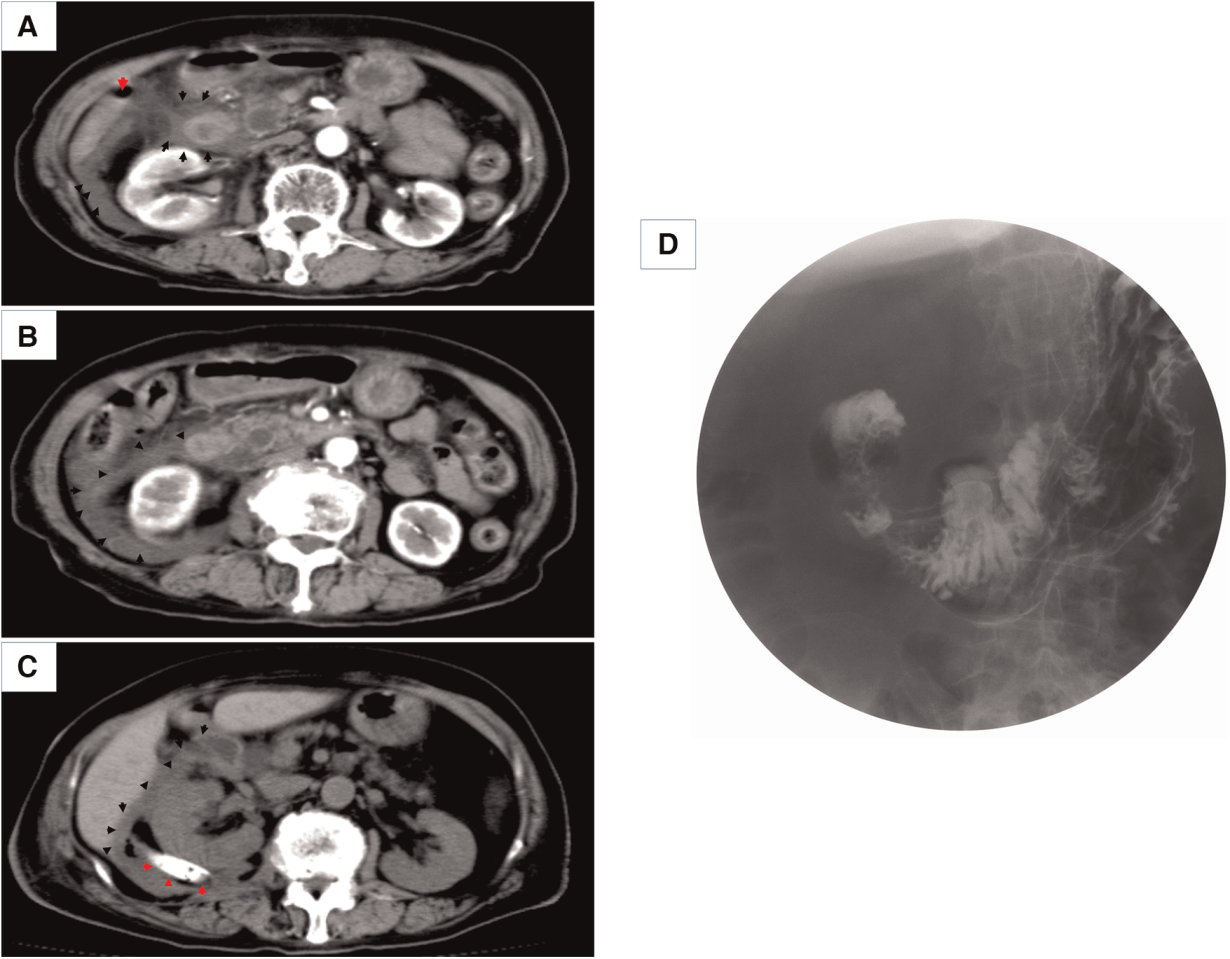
(A,B) Enhanced CT scans of the patient on admission and 7 days after conservative treatment (red arrows point to free abdominal gas, black arrows point to peri-duodenal and retroperitoneal exudate). (C) CT scan 7 days after percutaneous retroperitoneal tube placement for drainage (red arrows point to the placed retroperitoneal double cannula, black arrows point to periduodenal and retroperitoneal exudate). (D) shows a gastrointestinal barium study of the patient.
